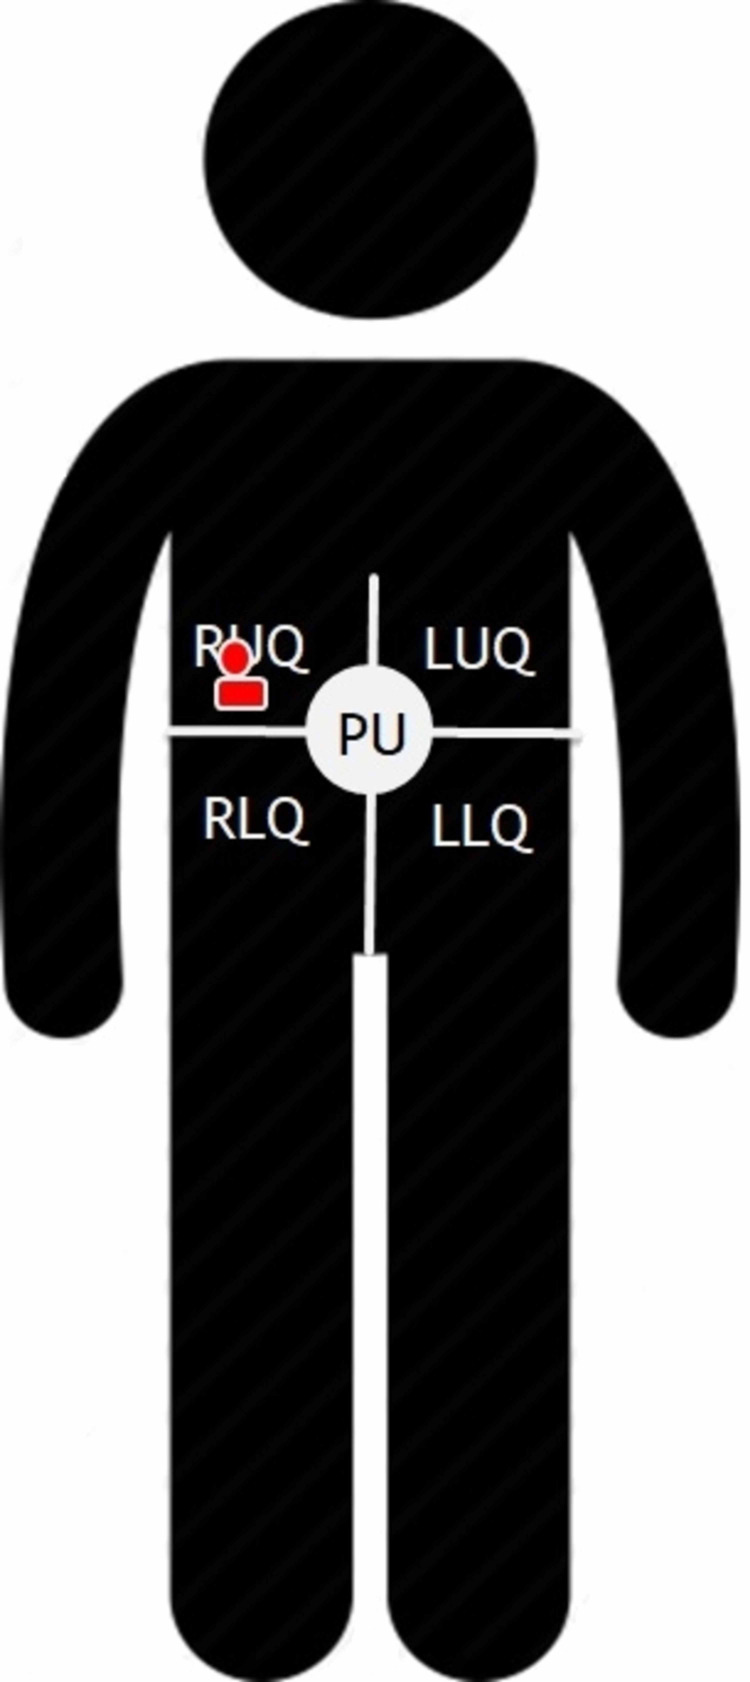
Positioning of stethoscope and ultrasound probe The abdomen was divided into five regions: right upper quadrant (RUQ), right lower quadrant (RLQ), left upper quadrant (LUQ), left lower quadrant (LLQ), periumbilical region (PUR).
